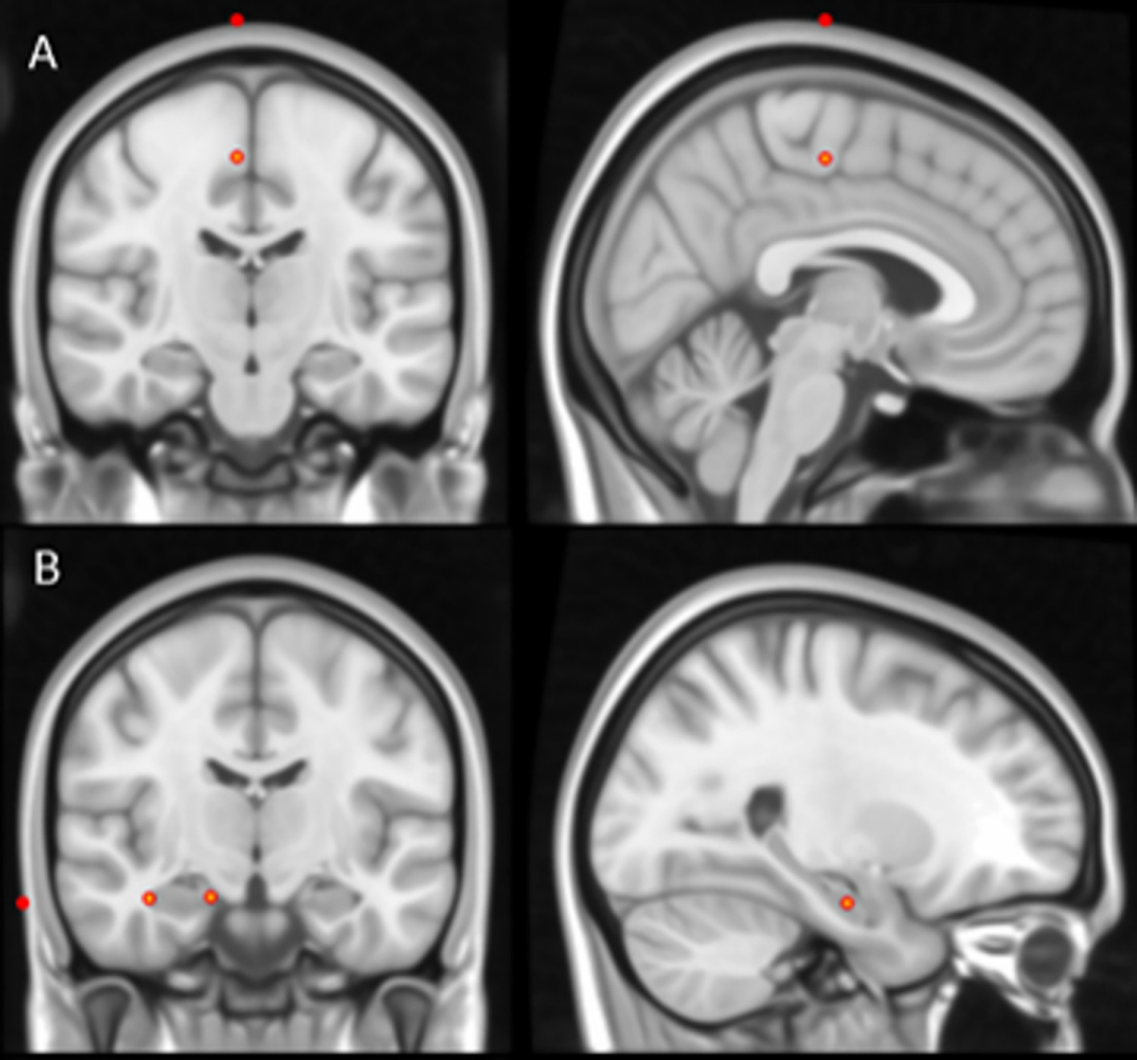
Key locations represented in coronal (left) and sagittal (right) view in MRI. A. Primary foot motor cortex location and the corresponding frontal surface location. B. Most distal and proximal points of the hippocampus and corresponding temporal surface location.
